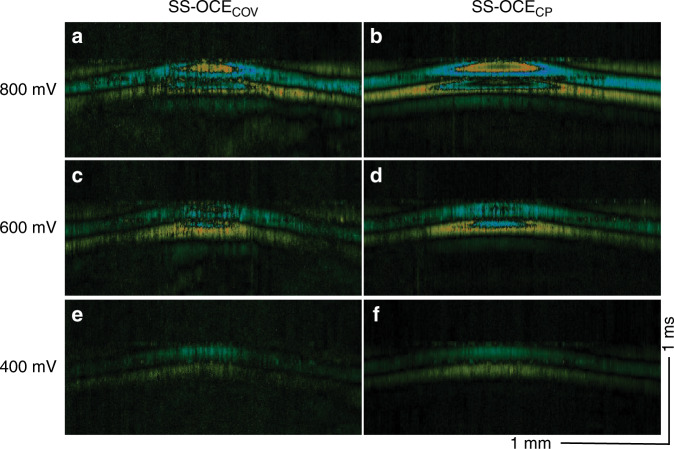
Spatiotemporal Doppler OCT of the cornea for different ARFs. a, c, e B-scans from the SS-OCE_COV_ system excited with large, medium, and small ARFs, respectively. (b), (d), and (f): B-scans from the SS-OCE_CP_ system excited with large, medium, and small ARFs, respectively
